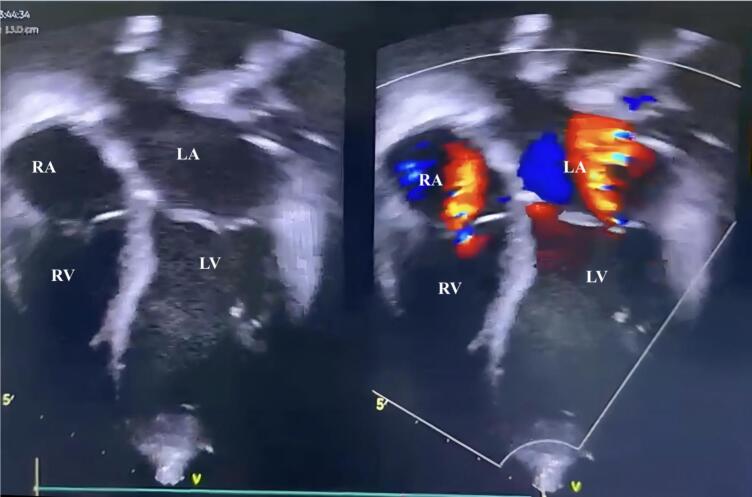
Postoperative echocardiography: complete removal of the tumor, no residual shunt from atrial septum. LA(left atrium); RA (right Atrium); LV (left ventricle); RV (right ventricle).
